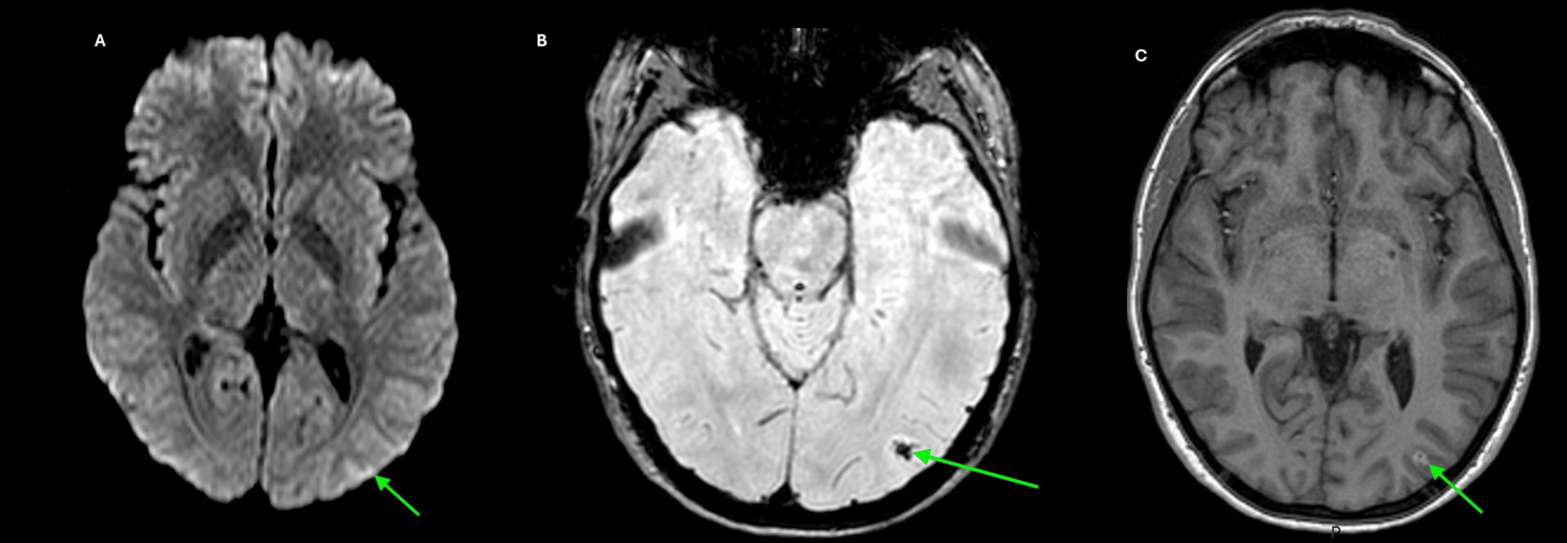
A follow-up brain MRI performed two weeks after the initial scan showing (A) near-complete resolution of the previously identified hyperintense lesion in the left parieto-occipital cortex (green arrow) on the diffusion-weighted sequence, (B) a susceptibility artifact in the left parieto-occipital cortex (green arrow), indicative of hemosiderin deposition and suggestive of prior hemorrhage on susceptibility-weighted imaging (SWI), and (C) cortical enhancement in the left parieto-occipital region (green arrow), supporting the presence of subacute hemorrhage on the post-contrast T1-weighted 3D fast field echo (FFE) MRI sequence.
